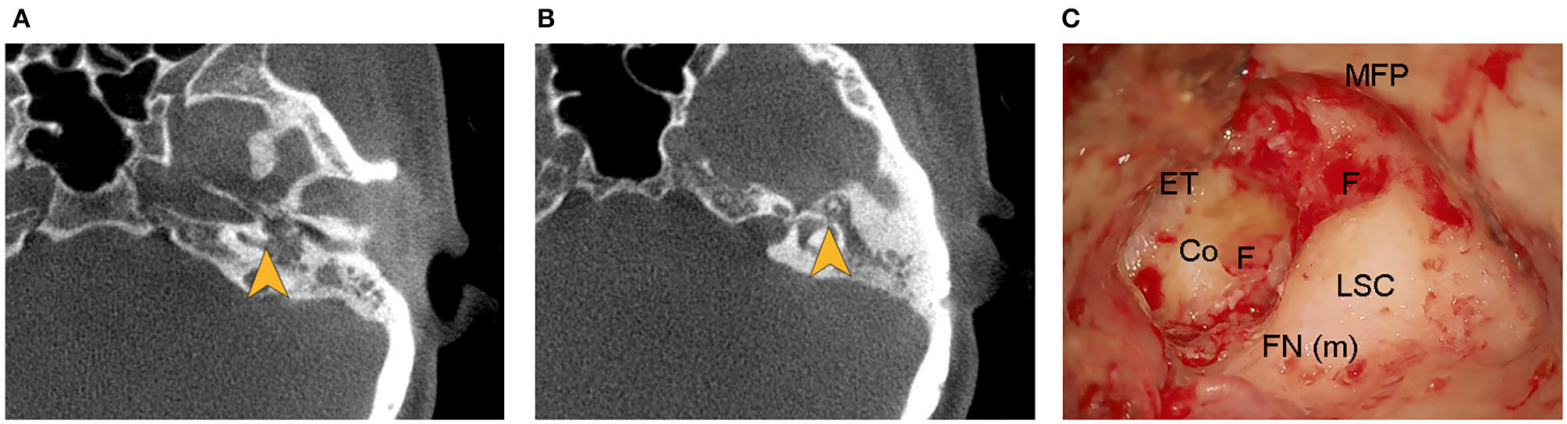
(A,B) Computed tomographic images of the temporal bone showed fistulae (yellow arrows) in both the basal turn of the cochlea and the horizontal semicircular canal. (C) Fistulae in both the basal turn of the cochlea and the horizontal semicircular canal were evident during the operation. Co, cochlea; ET, epitympanum; F, fistula; FN (m), mastoid segment of facial nerve; LSC, lateral semicircular canal; MFP, middle fossa plate.
